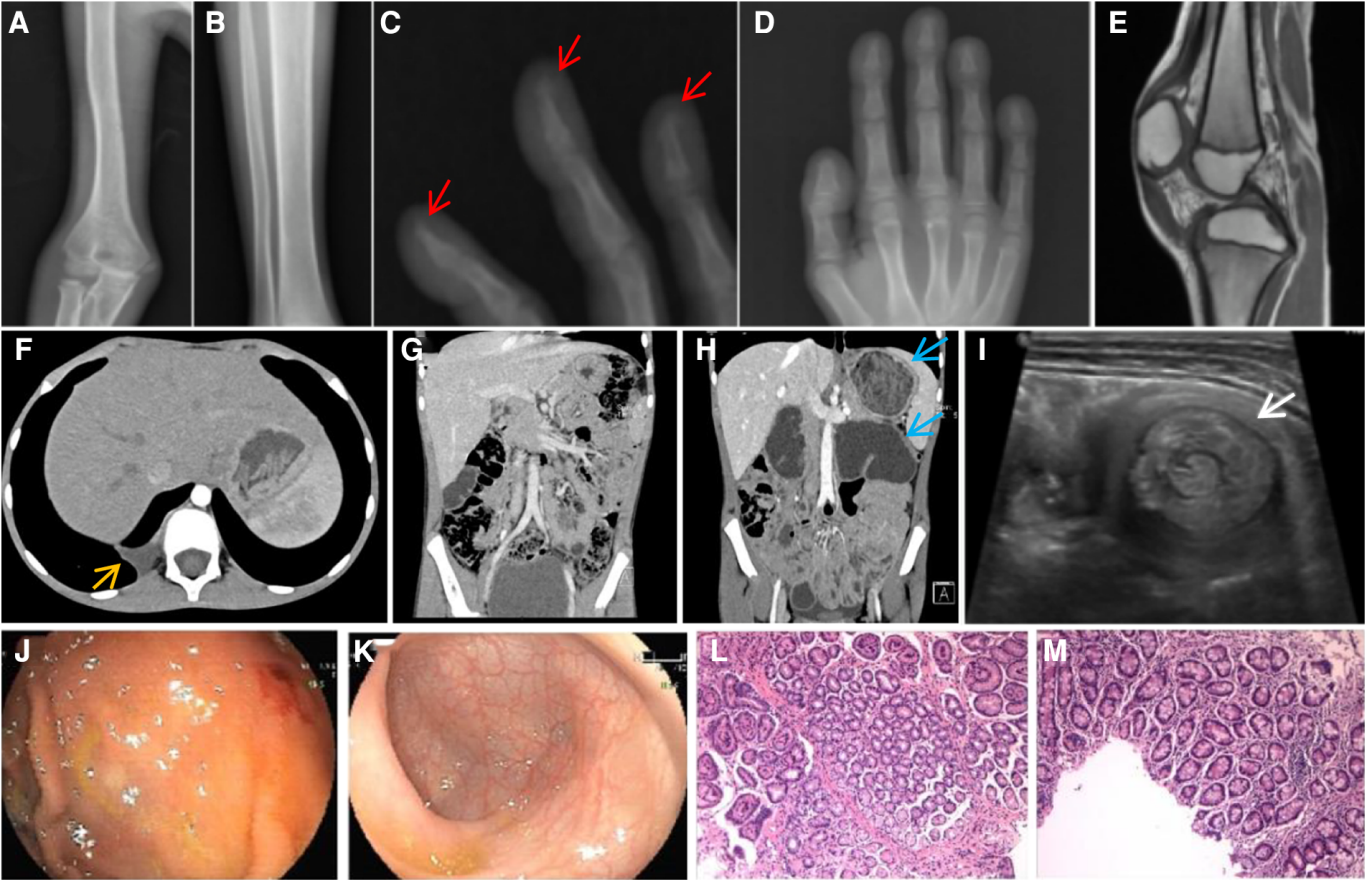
The long bone fragments of the child's hands, feet, and limbs show thickening of the periosteum, osteoporosis, and acro-osteolysis of the phalanges (arrow), swelling knee joint, and enlarged patellar bone with surrounding tissue hyperplasia (A–E). Lung computed tomography indicates local pleural thickening and traction (F). Compared with the results in 2019, food retention and duodenal dilation were remarkable by imaging in 2022 (G,H). Abdominal color ultrasound shows that the jejunum is lengthy, the local intestinal wall is thickened, and it gathers into a mass. There is a transcendental intussusception, which can be unzipped by itself (I). Electronic gastroenteroscopy revealed a rough gastric mucosa (J) and smooth colonic mucosa (K). Pathological biopsy of gastric and intestinal mucosal tissues showed mild changes in chronic inflammation (L,M).
